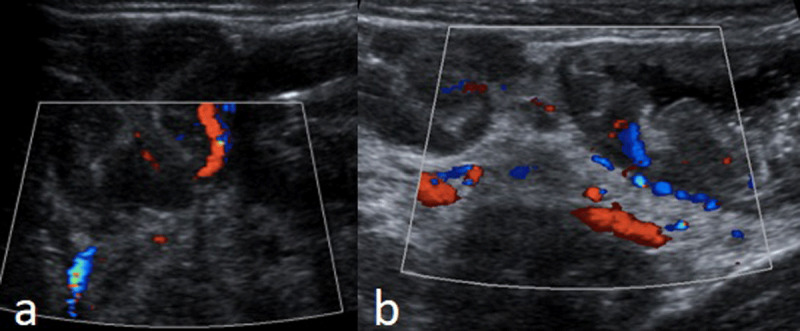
Abdominal ultrasound – diffuse parietal thickening of the colon, with increased vascularization on color Doppler evaluation. The mesentery at the right upper quadrant (b) was also thickened and showed increased vascularization.
